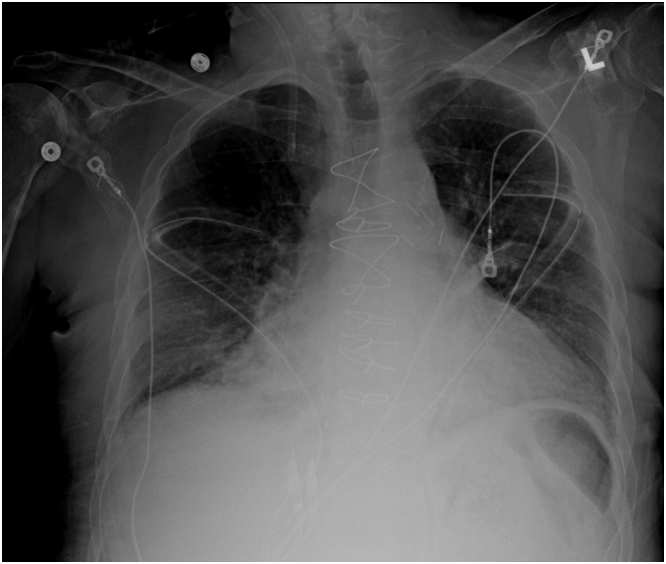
Postoperative CXR With the TEPW In Situ Postoperative chest x-ray of the patient showing no evidence of migrated temporary epicardial pacing wires.
